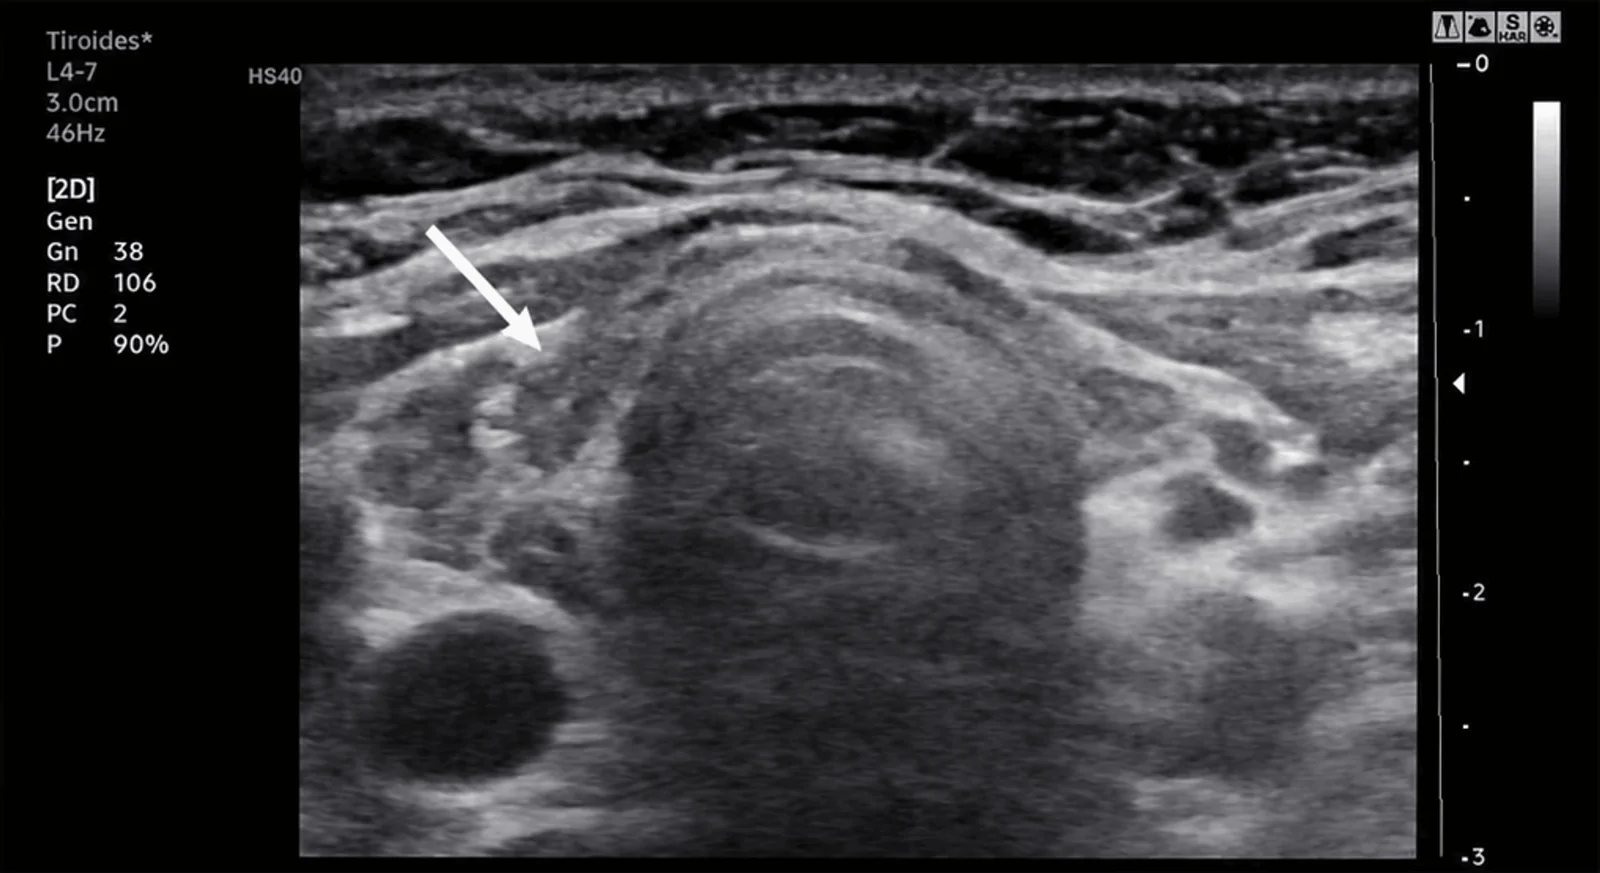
Thyroid ultrasound consistent with chronic thyroiditis Thyroid ultrasound obtained from the patient presented in this case. The arrow indicates the heterogeneous thyroid parenchyma consistent with chronic thyroiditis
